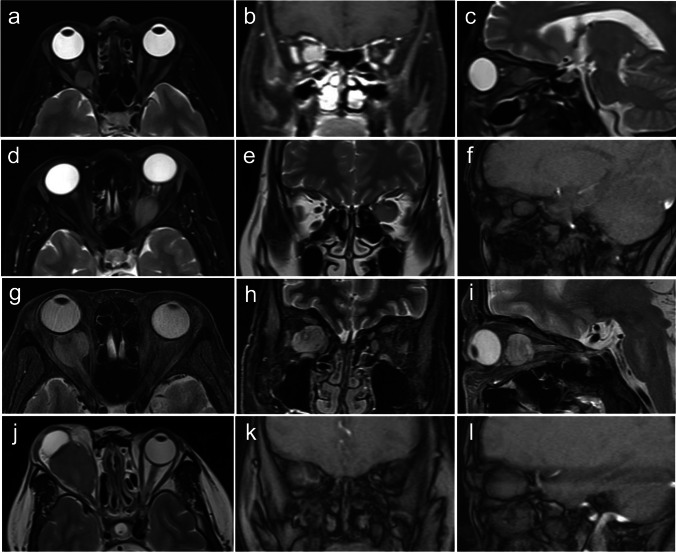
Four cases of intraorbital optic nerve sheath meningiomas (ONSMs). Preoperative magnetic resonance imaging (MRI) showed the location of tumors and surgical considerations. a–c Case 6 MRI with gadolinium (Gd) enhancement which underwent endoscopic transnasal approach (ETA) only. a Axial T2-weighted MR image; b coronal T1-weighted MR image; c sagittal T2-weighted MR image. d–f, g–i, and j–l Case 20, case 4, and case 24 MR images with/without Gd enhancement which underwent ETA combined with transconjunctival approach (TCA). d Axial T2-weighted MR image with Gd enhancement; e, f coronal and sagittal T2-weighted MR image; g–i axial, coronal and sagittal T2-weighted MR image; j axial T2-weighted MR image; k, l coronal and sagittal T1-weighted MR image
